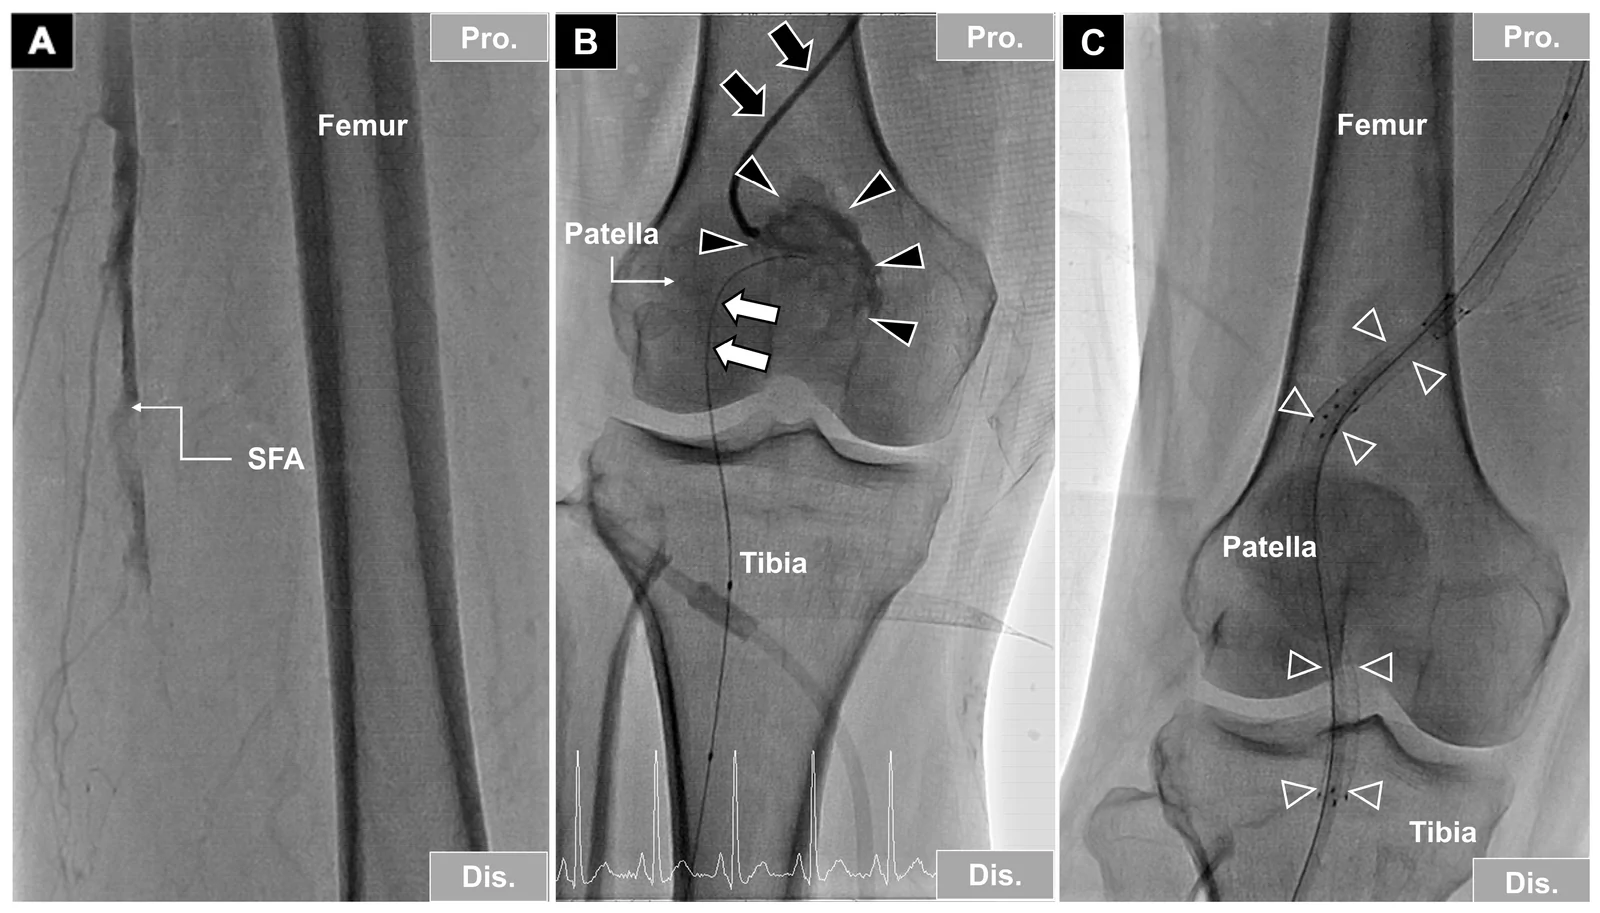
Digital subtraction angiography (DSA) and endovascular reconstruction of the thrombosed popliteal artery aneurysm. Panel (A) shows chronic total occlusion of the distal superficial femoral artery (SFA) with abrupt termination proximal to the thrombosed aneurysmal segment. The aneurysmal sac shows no contrast opacification (black arrowheads), which confirms its complete exclusion from active luminal flow. This lack of contrast opacification correlates with the heterogeneous, thrombus-filled sac observed on prior ultrasound and magnetic resonance imaging (MRI). Panel (B) shows an endovascular catheter (white-outlined black arrow) advanced antegrade from the SFA toward the occluded native popliteal artery (white arrows). Unlike non-invasive modalities, catheter-based angiography directly delineates the inflow obstruction, collateral networks, and distal runoff to guide endovascular reconstruction [4,8]. A further consideration was that open exposure would have required extensive dissection around the large, thrombosed sac. Endovascular repair was nevertheless selected in this patient, because the presentation was one of symptomatic chronic popliteal occlusion and the anatomy was suitable for a minimally invasive approach. Open exposure would also have required extensive dissection around the large, thrombosed sac. This sac lay directly adjacent to the tibial nerve and popliteal vein, and such dissection was expected to increase surgical morbidity. Contemporary guidelines and comparative studies support endovascular repair as a reasonable alternative in appropriately selected patients [4,9,10,11]. Panel (C) shows two overlapping self-expanding Viabahn covered stent grafts (white arrowheads) deployed after successful traversal of the occluded femoropopliteal segment. Together they exclude the aneurysm and restore arterial continuity. To support long-term vascular patency after Viabahn covered stent-graft placement, the patient received dual antiplatelet therapy comprising aspirin 100 mg/day and clopidogrel 75 mg/day for 6 months, followed by lifelong aspirin 100 mg/day monotherapy. This regimen was prescribed in accordance with current guideline recommendations for lower extremity peripheral artery disease [9]. Abbreviations: Dis., distal; Pro., proximal.
